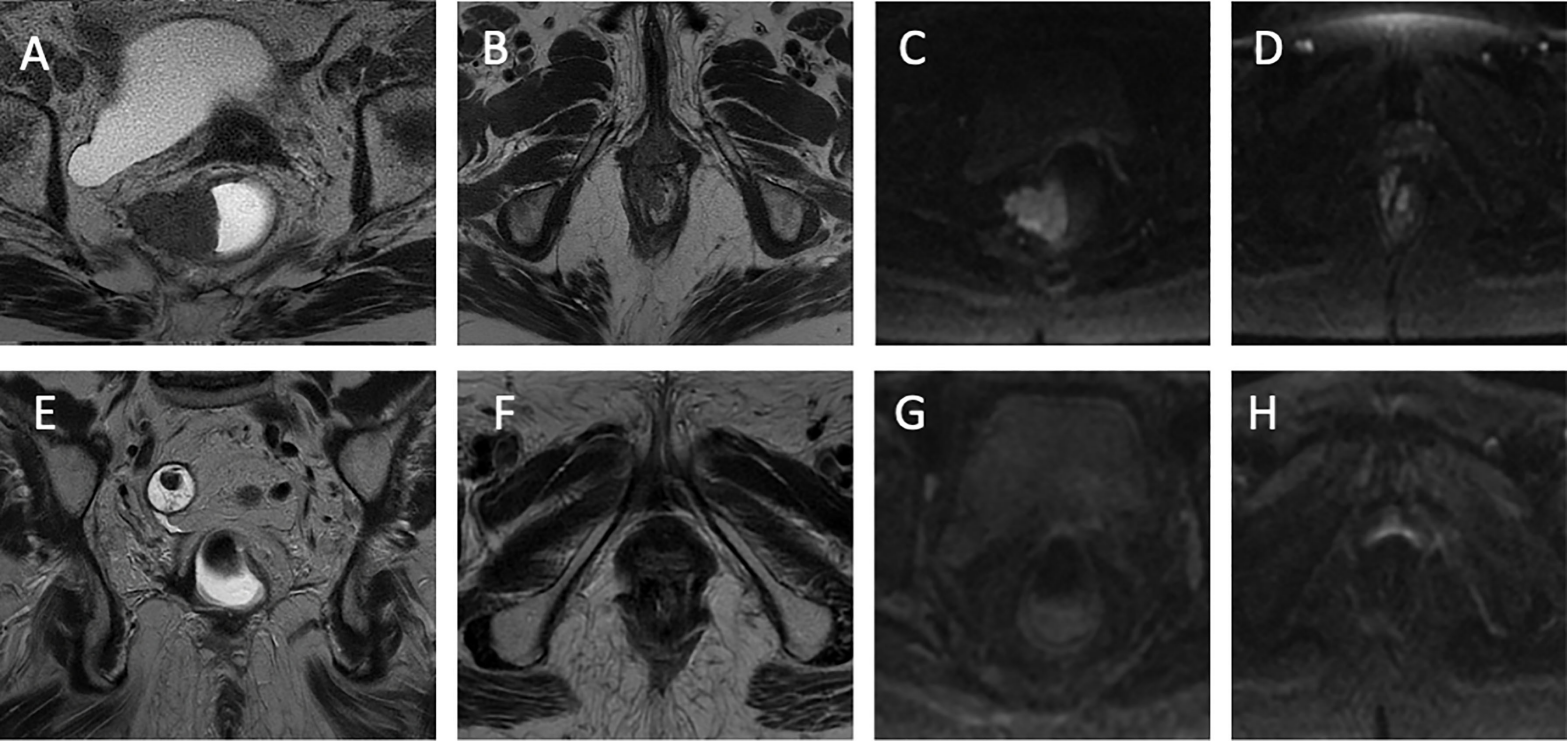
Radiologic images. (A) The T2-weighted sequence of rectal cancer on the axial plane. Parietal neoplastic formation of the middle rectum with a polypoid appearance and a broad implant base is observed on the right posterolateral wall from 6 to 12 o’clock, which causes the narrowing of the rectal lumen. This rectal formation infiltrates the mesorectum until it exceeds the mesorectal fascia by about 4.5 mm and infiltrates the elevator muscle. (B) The T2-weighted sequence of anal cancer on the axial plane. At the level of the rectal junction at 11 o’clock, there is evidence of neoplastic tissue infiltrating the internal and external anal sphincter. (C) Sequence weighted in DWI in correspondence with the rectal lesion. Signal restriction in the DWI sequence. (D) Sequence weighted in DWI at the level of the anal canal. Signal restriction in the DWI sequence. (E) Coronal plane T2-weighted sequence. A clear hypointensity is observed with regard to fibrotic outcomes 6 months after the start of radiochemotherapy (CRT). (F) T2-weighted sequence on the axial plane. Anal lesion response after CRT. (G) Sequence weighted in DWI in correspondence with the rectal lesion. No signal restriction following CRT. (H) Sequence weighted in DWI at the level of the anal canal. No signal restriction following CRT.
